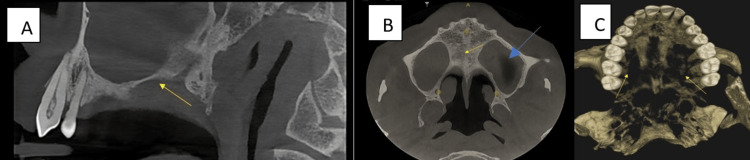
CBCT A. Sagittal section revealed no erosive changes and intact floor of the maxillary sinus (yellow arrow). B. Axial section revealed increased mucosal thickening within the left maxillary sinus (blue arrow) and no erosive changes on the hard palate (yellow arrow). C. 3D-reconstructed CBCT revealed a bone loss in the posterior maxillary quadrants
